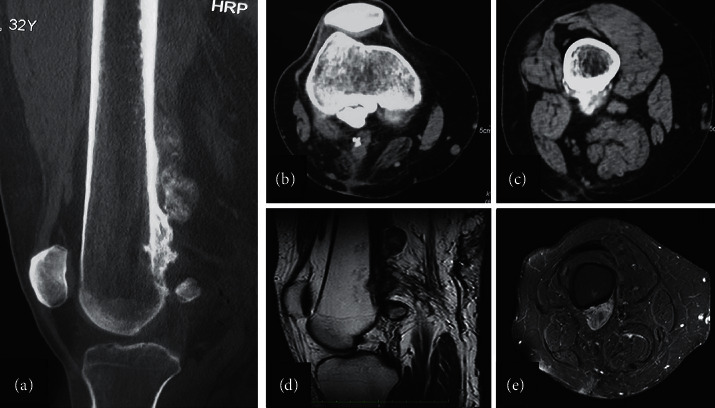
Computer tomography (CT) of the right femur in a 32-year-old male with parosteal osteosarcoma of the distal femur (a). Sagittal CT with multiple large-ossified masses adjacent to the posterior surface of the distal femur (b). Axial view displaying the tumor's broad-based stuck attached to cortical surface associated with dense central ossification and the cleft sign that separated the ground glass mass from the bone cortex, with no evidence of medullary involvement (c). Magnetic resonance imaging of the distal femur (sagittal (d) and axial (e) views) showing the round mass of low signal intensity on T1-weighted imaging. The cortex appears intact along the deep surface of the lesion.
